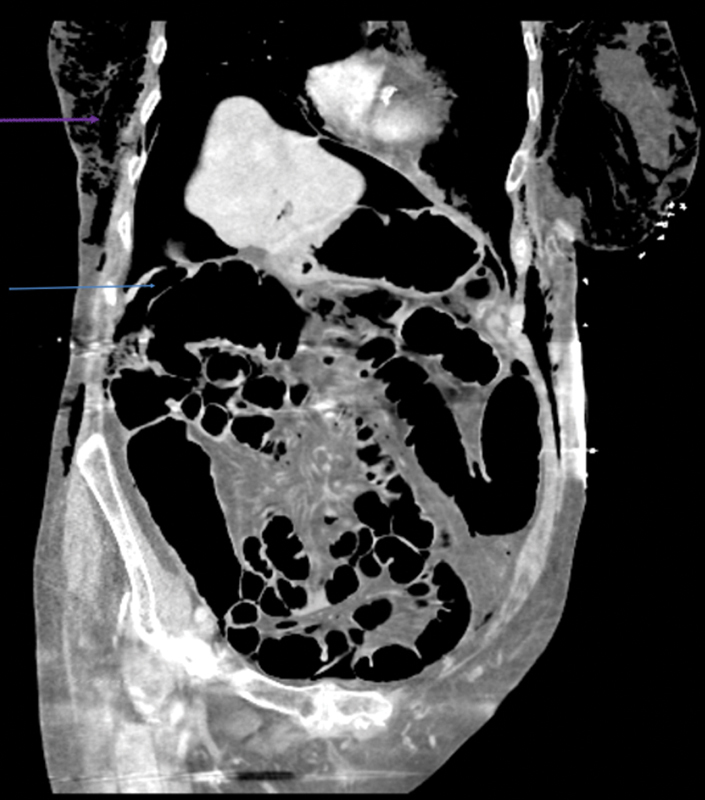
Coronal computed tomography image demonstrating free air in the peritoneal cavity (blue arrow) and subcutaneous emphysema in the thoracic and abdominal walls (purple arrow).
